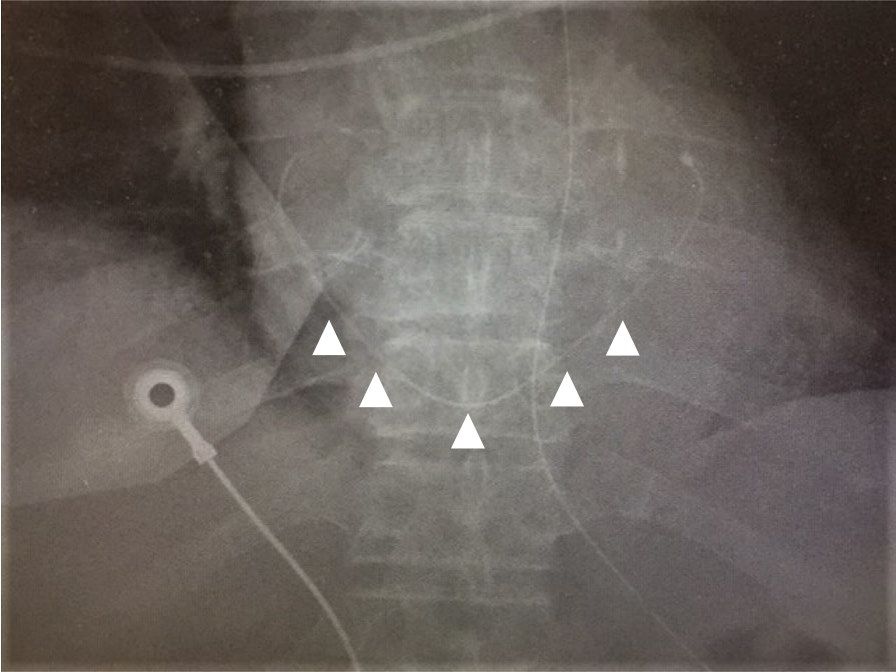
A close examination of the chest X-ray (Fig. 1) taken immediately after cardiopulmonary resuscitation indicated a severed catheter (arrows)
